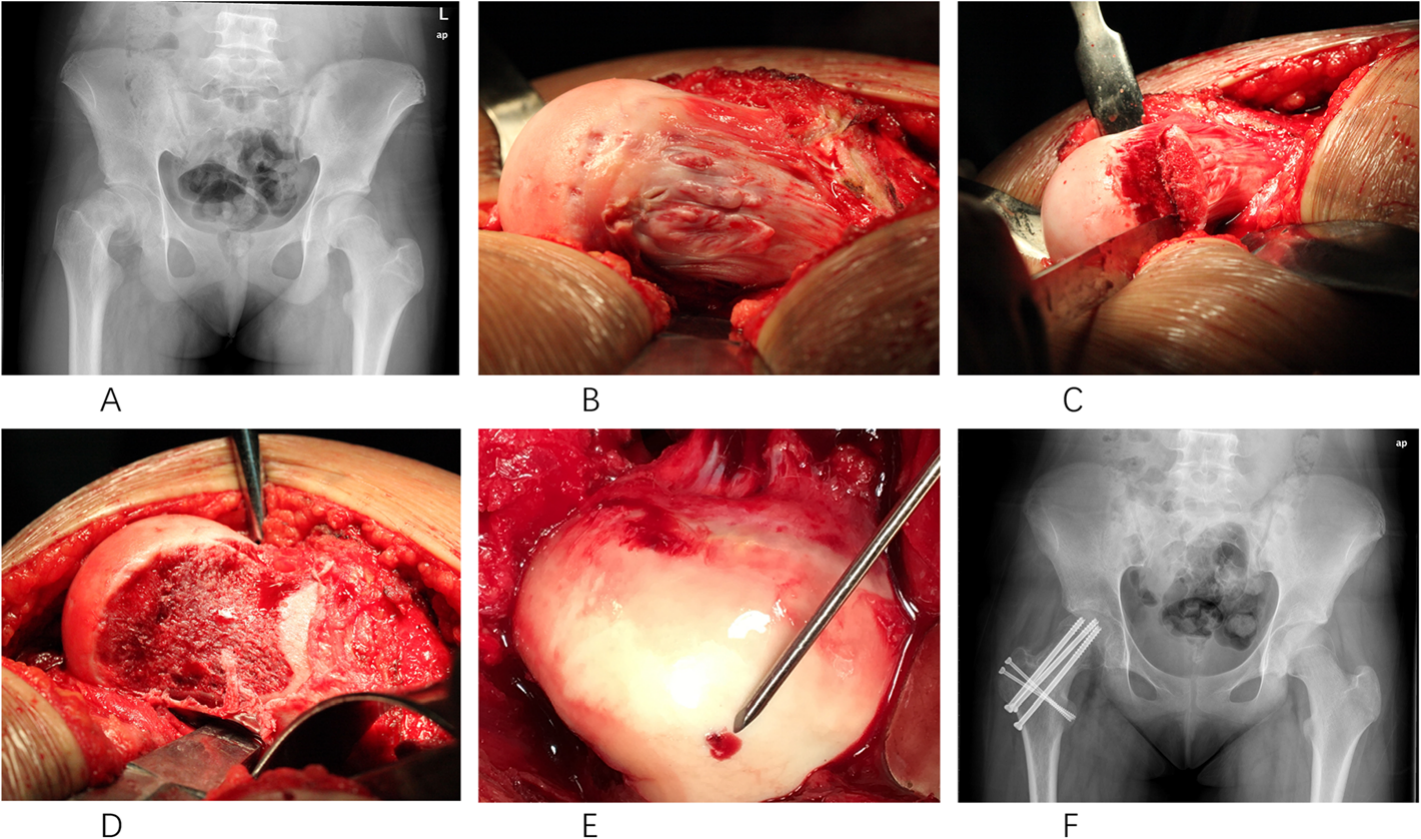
A 12-year-old female patient presented with pain in the right hip. a Anteroposterior (AP) radiograph demonstrates severe posteromedial displacement of the capital femoral epiphysis. b Intraoperative picture shows the displacement. c Modified Dunn osteotomy after surgical hip dislocation (SHD). d Femoral osteochondroplasty was performed to restore the proximal femoral anatomy and femoral head sphericity. e Positive bleeding sign after reduction. f Postoperative AP view at 1-year follow-up with excellent hip motion
